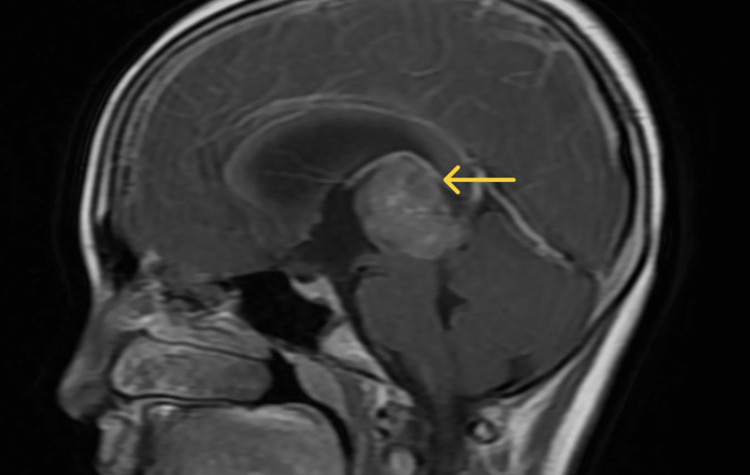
Postcontrast sagittal MRI shows significant heterogenous contrast uptake by the lesion. MRI, magnetic resonance imaging
